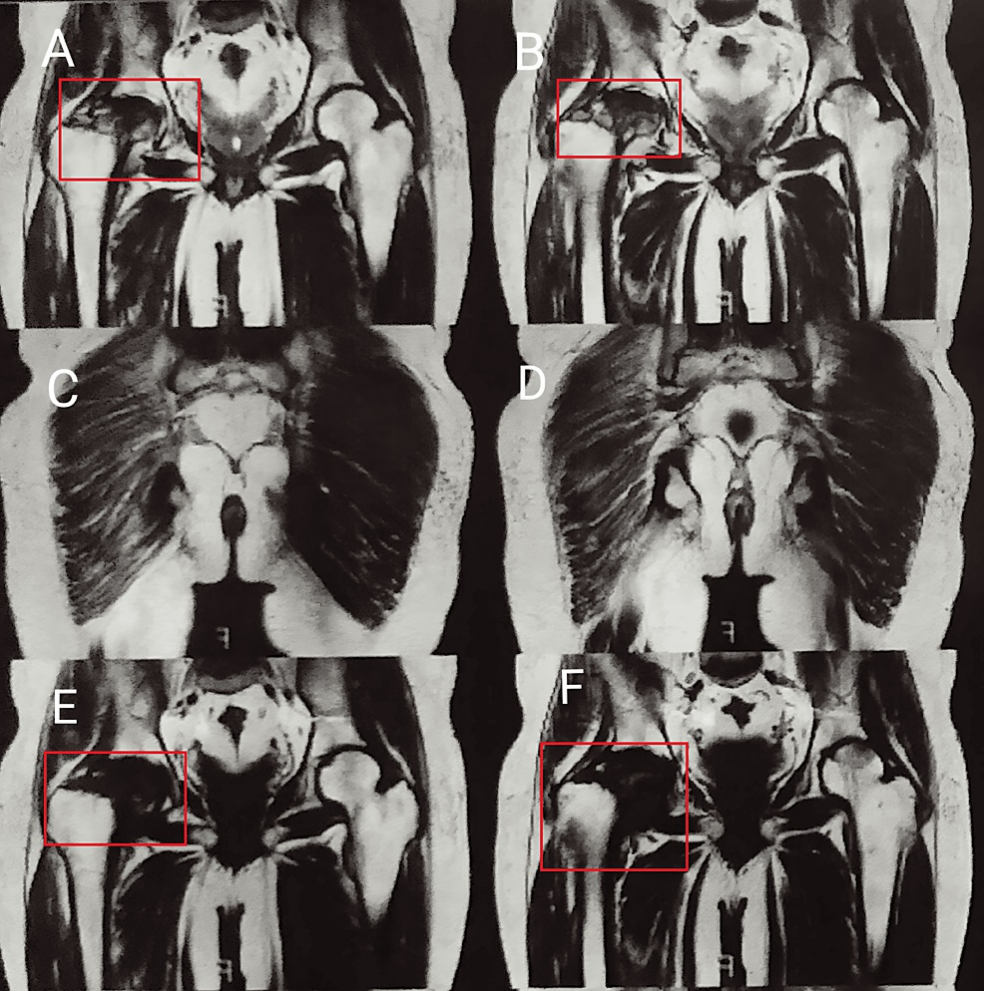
(A-F) MRI of the hip joint in which the boxes on images A, B, E, and F indicate avascular necrosis of the hip (Grade III)
